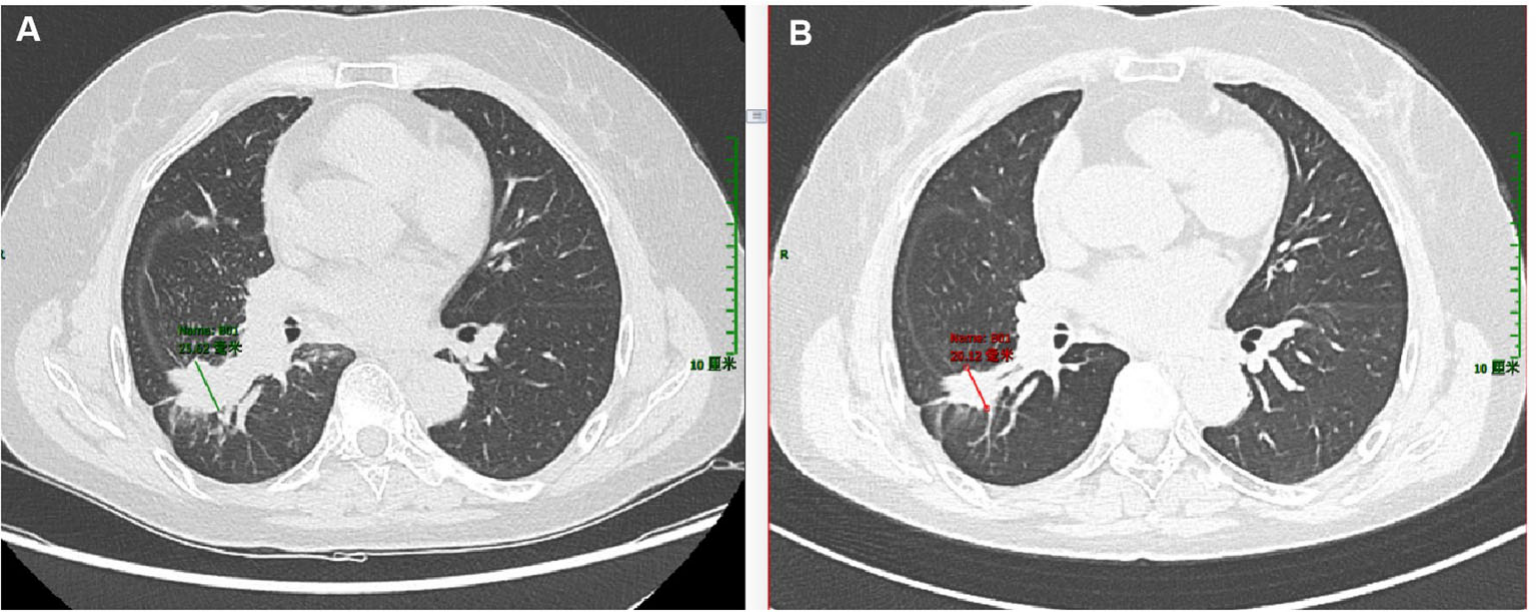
Enhanced computed tomography (CT) images of the lungs of the second patient. (A) CT scan of the lungs taken upon admission in April 2024. (B) CT scan of the lungs re-performed in November 2024.
